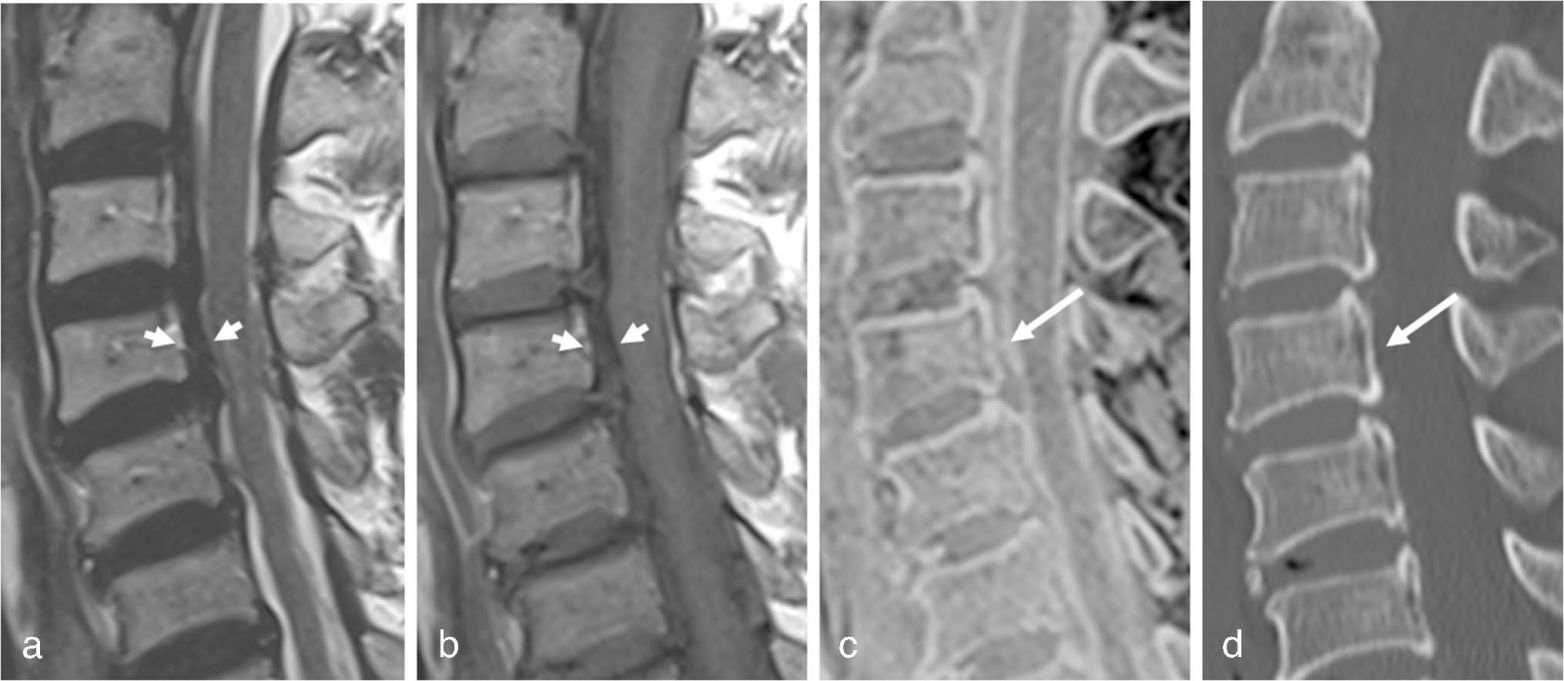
Eighty-two-year-old male who presented with weakness in both lower extremities. On sagittal T2- and T1-WI (a and b), a low-signal intensity band is seen between the posterior wall of the C4 vertebral body and dural sac (short arrows), mimicking calcified PLL. However, no OPLL was observed on 3D FLASH, which was confirmed by CT (arrows, c and d)
